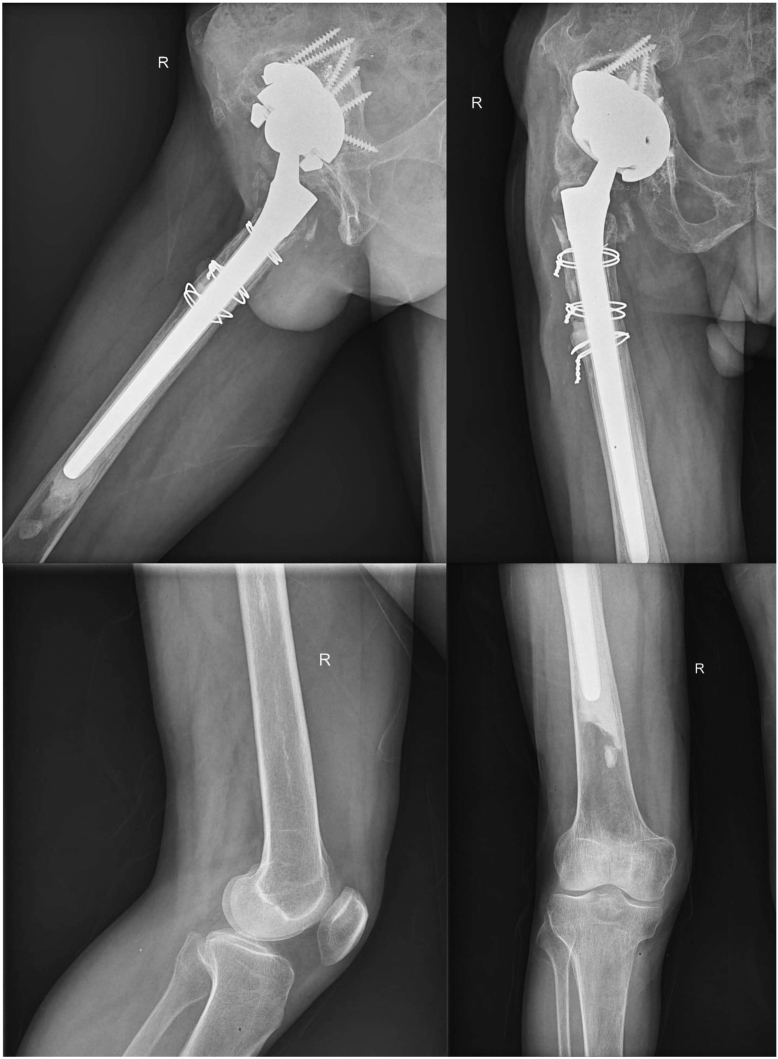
Final follow-up radiograph of case 2.
